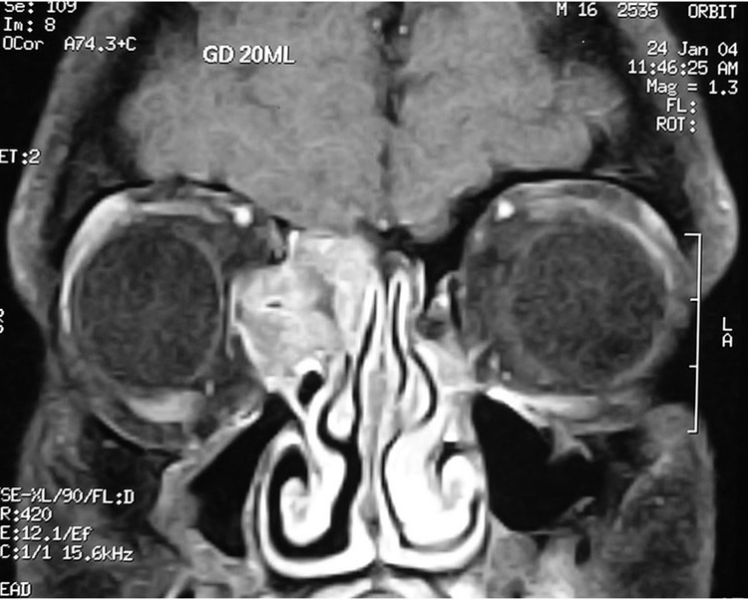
Preoperative MRI scan. Coronal T_1_W post-contrast image MRI scan, showing a mass filling the anterior portion of the right ethmoid air cells. The mass demonstrates patchy enhancement, protrudes into the adjacent orbit, depicts soft tissue intensity, and enhances following contrast administration.
